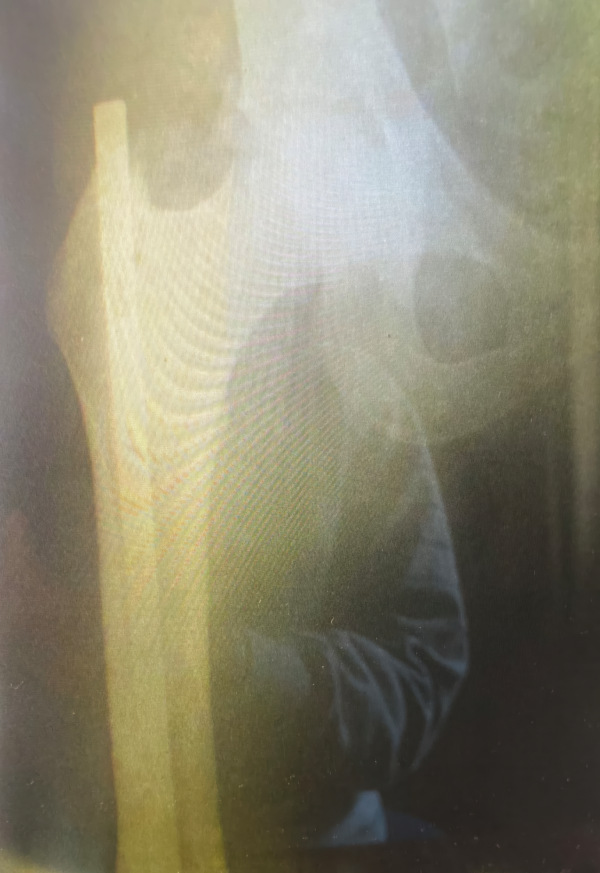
X-ray of Ukrainian Claimant Submitted to German Authorities for Reparations. The femur (thigh bone) with the Küntscher intramedullary nail is seen; there are no signs of callus or a healed fracture line, despite the poor quality of the photograph. Photo provided courtesy of M. Dubyk.
